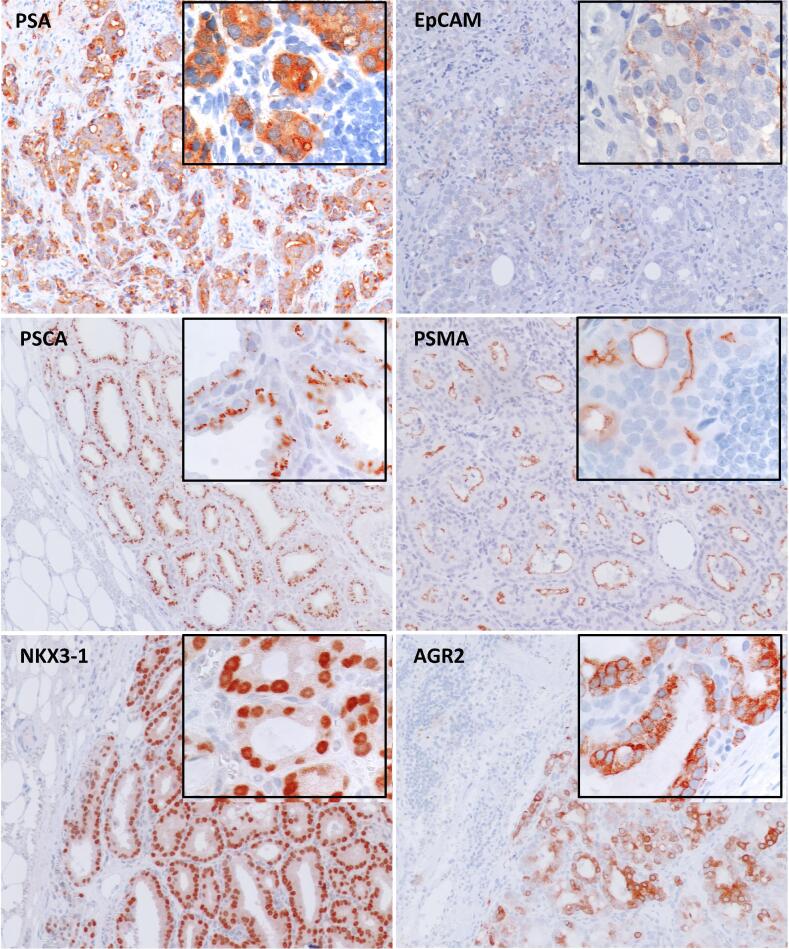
Protein expression of lymph node section from prostate cancer patients with macroscopic metastases (pN1) at 10× and 60× magnification (insert). PSA = prostate-specific antigen; PSCA = prostate stem cell antigen; PSMA = prostate-specific membrane antigen.
